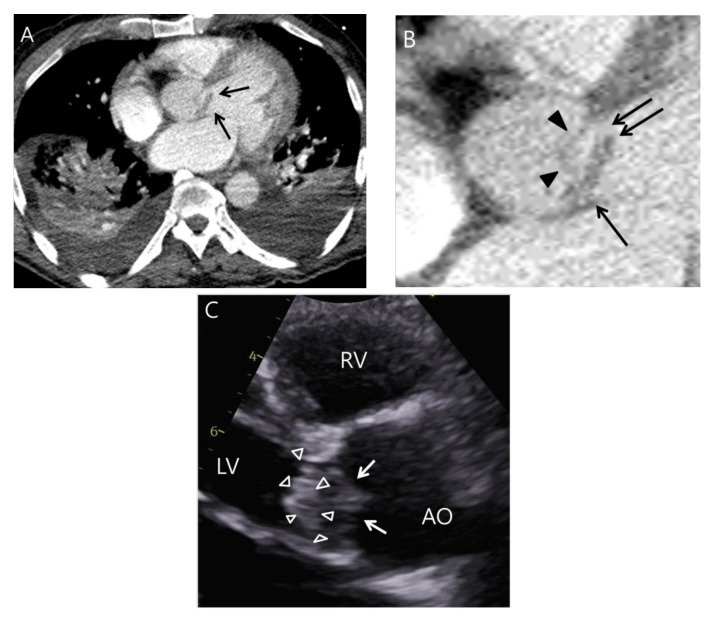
A case of the indirect-oscillation sign on routine chest CT. A 62-year-old man presented to the ED with acute dyspnea. (A) Note the area of elongated nodular thickening attached to the aortic valve on an axial CT image. (B) On the magnified view, the elongated nodular thickening is clearly different than the normal aortic valve cusp (arrowheads) because it has a blind end (double arrows) with an abnormal orientation. This CT finding is considered indicative of IE on routine chest CT (i.e., indirectly reflecting oscillation). (C) Subsequent transthoracic echocardiography (TTE) revealed an echogenic vegetation with elongated appearance (arrowheads) attached to the aortic valve (arrows). LV, RV, and AO indicate left ventricle, right ventricle, and aorta, respectively.
